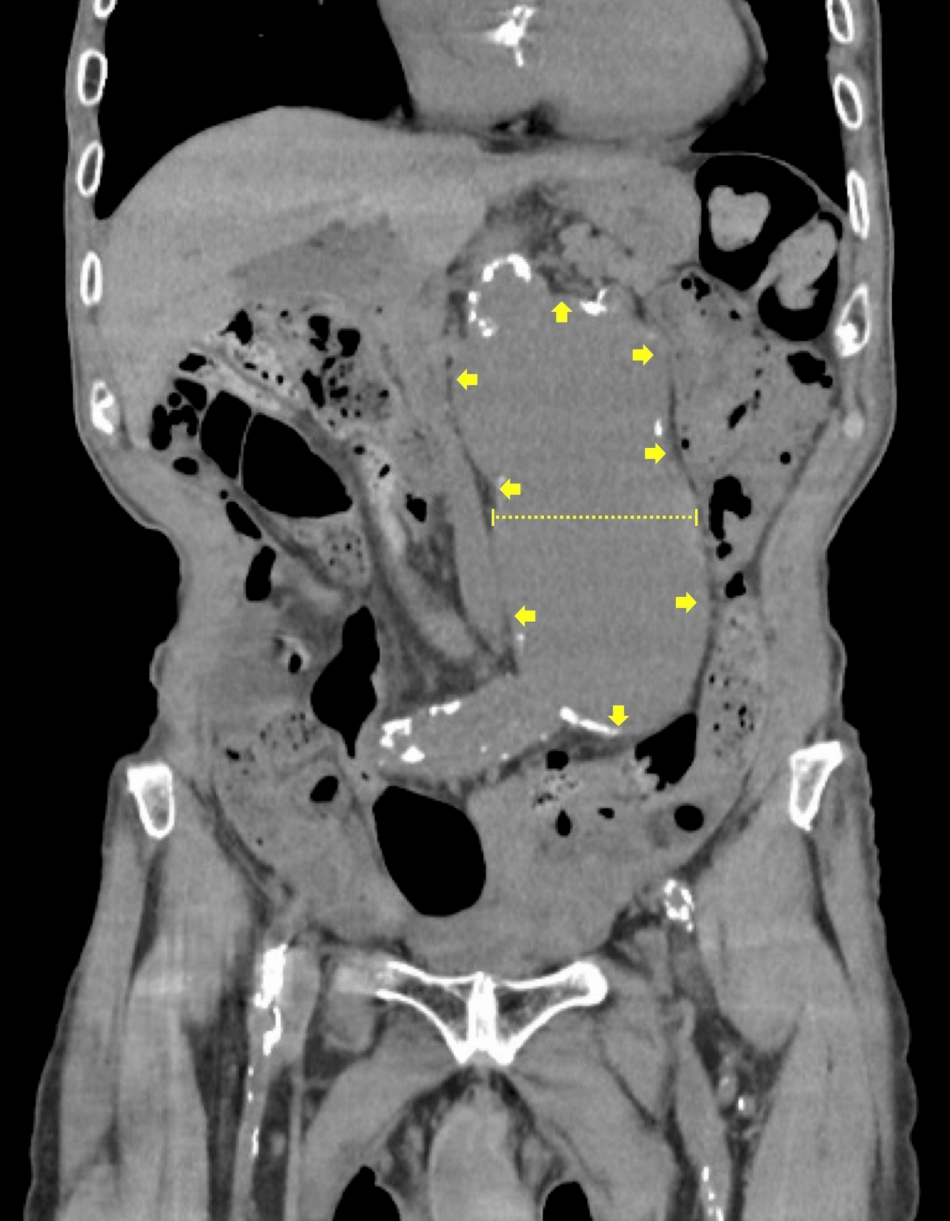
Abdominal CT showing a large aortic aneurysm. Coronal abdominal CT image taken 18 days prior to admission, demonstrating a large abdominal aortic aneurysm (arrows) with a diameter of 68.8 mm.
